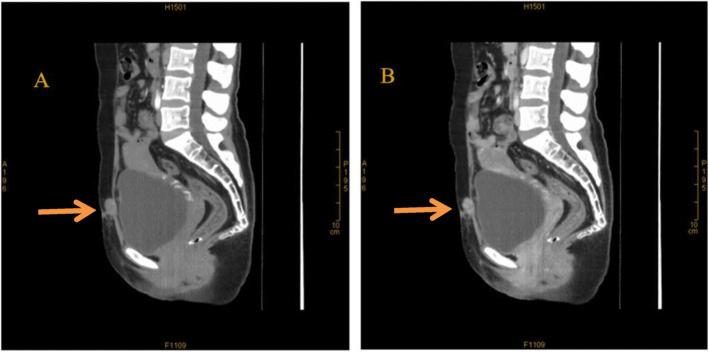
Computed tomography scan of the pelvis showing several nodules in the abdominal wall midline fascia (indicated by the arrows). The largest nodule was approximately 21 × 15 mm in size, with clear boundaries but less uniform in internal echoes. (a) Arterial phase enhancement, nodules mildly enhanced. (b) Venous phase enhancement, nodules further enhanced
